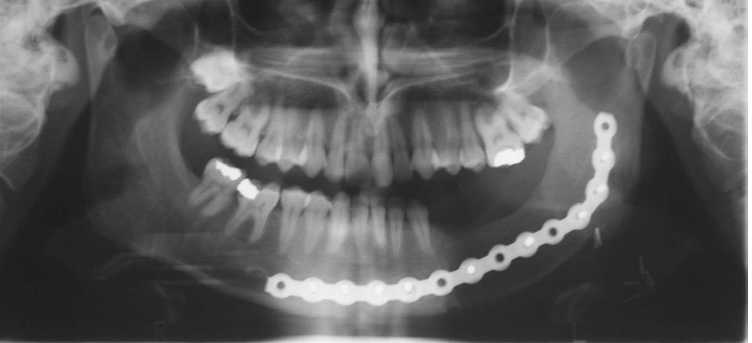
panoramic radiograph of patient n°1, six months after surgery.
